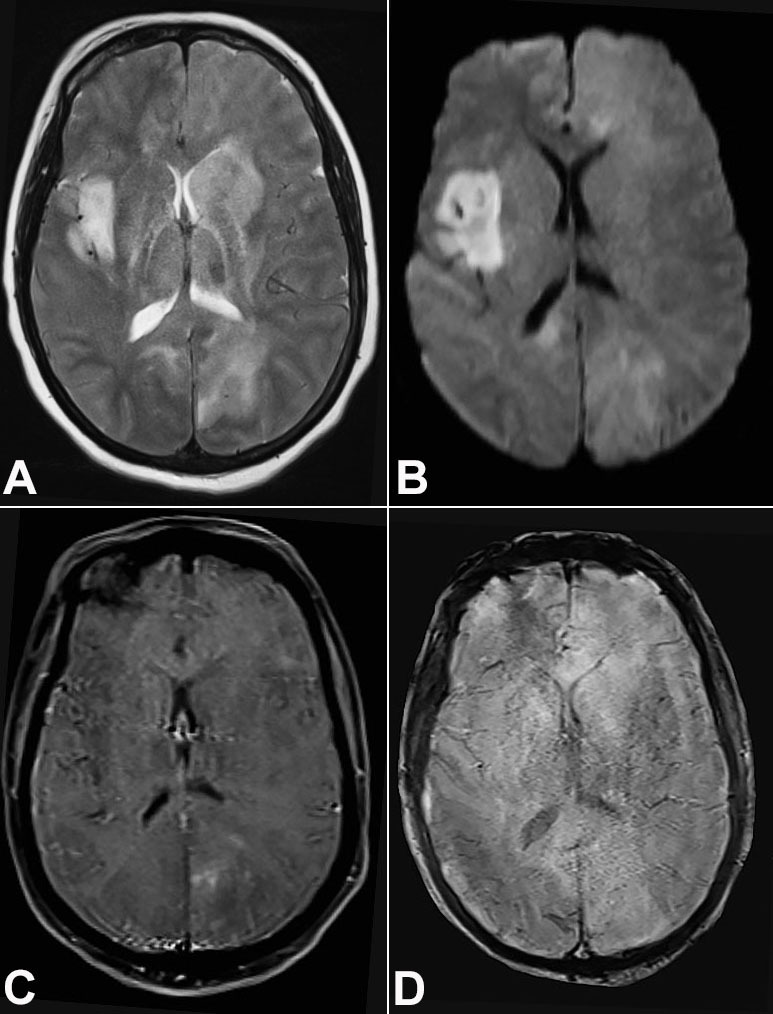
Brian MRI. A – Axial T2 weighted image shows ill-defined hyperintense signal involving bilateral basal ganglia (Left > Right), thalami, right insula, cortical and subcortical white matter in bilateral occipital lobes (Left> Right); B – Axial Diffusion weighted image shows areas of patchy diffusion restriction, more in the right insula; C – Axial T1 weighted post contrast image shows areas of mild patchy left occipital enhancement; D – Axial SWI image shows no evidence of hemorrhage
